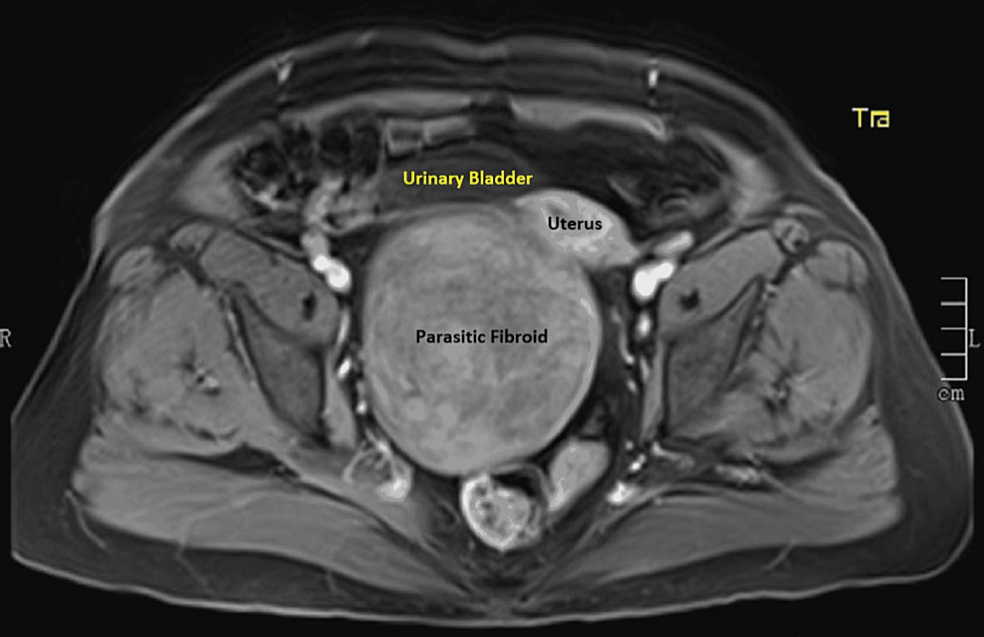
MRI of the abdomen and pelvis (T1-weighted axial image) showing parasitic fibroid, uterus, and urinary bladder. MRI: magnetic resonance imaging
